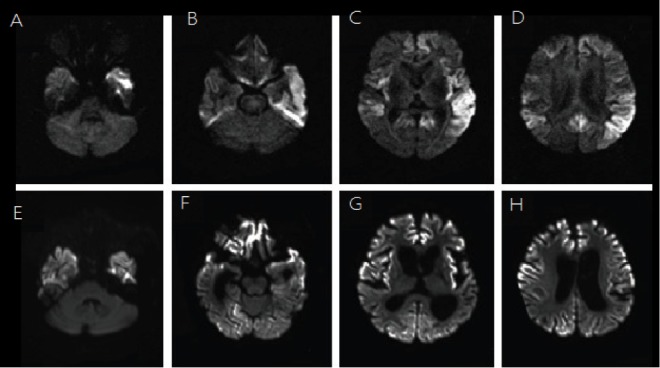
MRI brain [A-H] Shows persistent diffusion abnormality involving the lentiform nucleus on the left, with mild abnormality involving the lentiform nucleus on the right and diffusion abnormality in the cortex of the cerebral hemispheres, left greater than right
